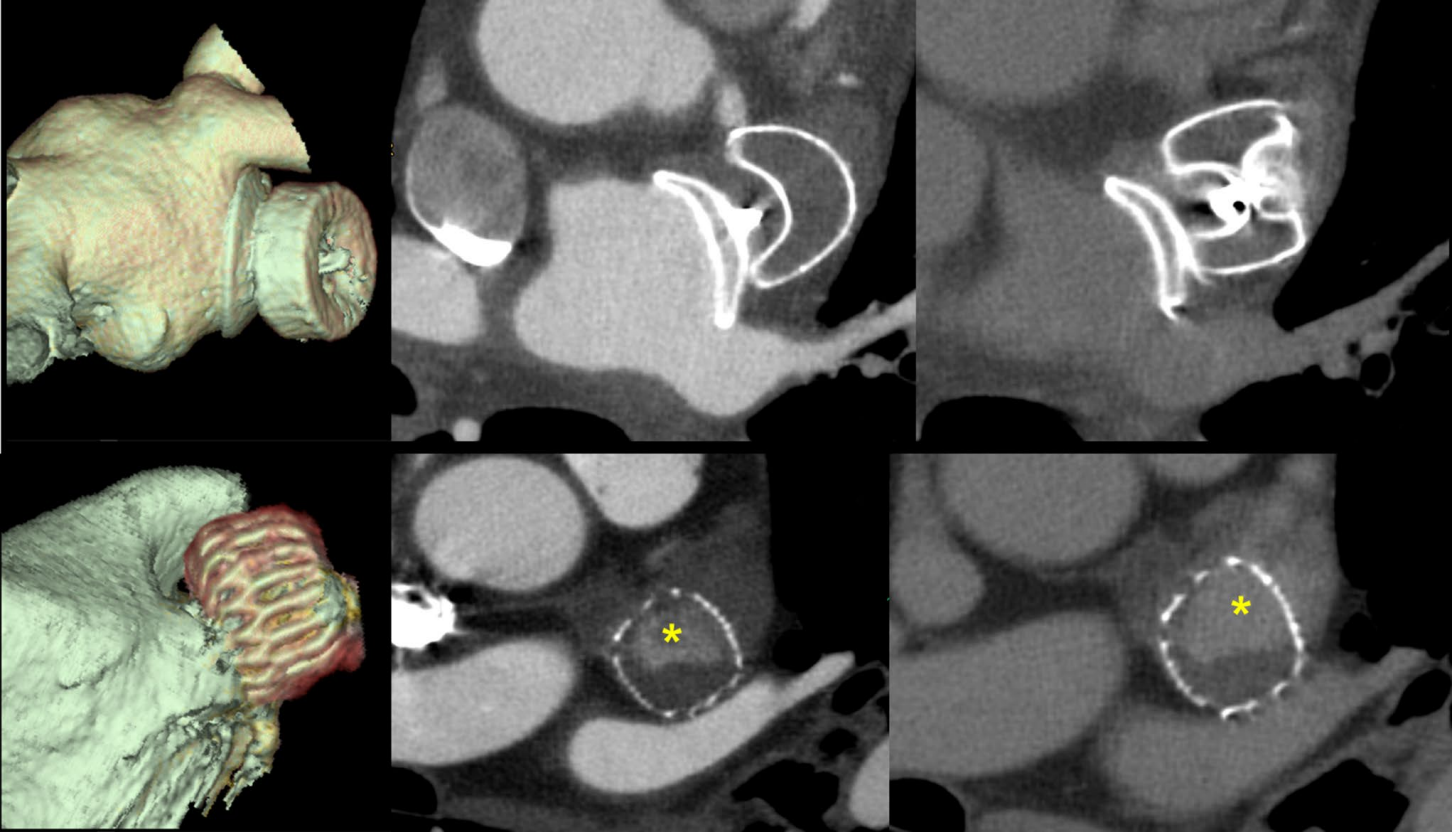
Post LAAO assessment using CCT. Upper panel depicts an Amplatzer Amulet 31 mm occluder device with completely thrombosed LAA, evident by the lack of perfusion in the arterial and delayed imaging phase (from left to right). Lower panel depicts a Watchman FLX 24 mm occluder device with rest perfusion (asterisk) of the only partially thrombosed LAA, in both the arterial and delayed imaging phase (from left to right). LAAO; left atrial appendage occlusion, LAA; left atrial appendage, CCT cardiac computed tomography
